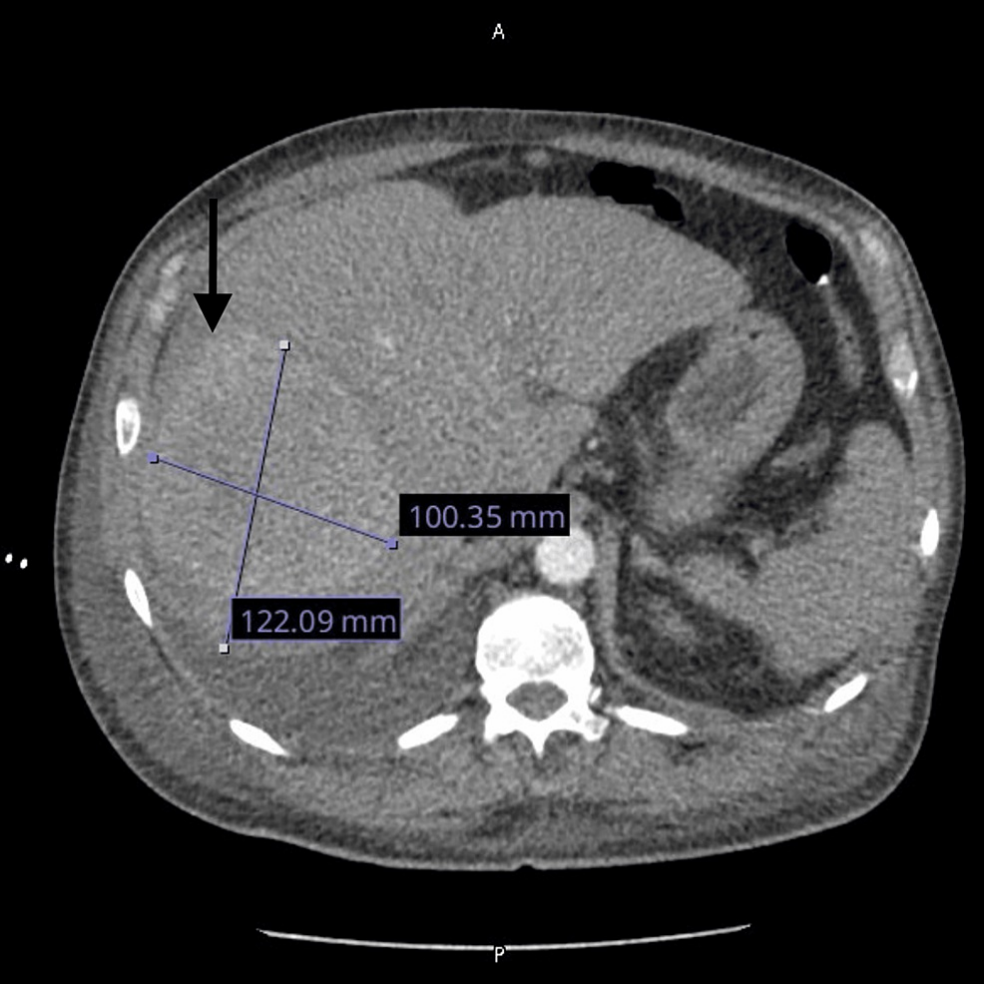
Hyperdense liver mass Computed tomography (CT) of 10.04 x 12.21 cm liver mass
